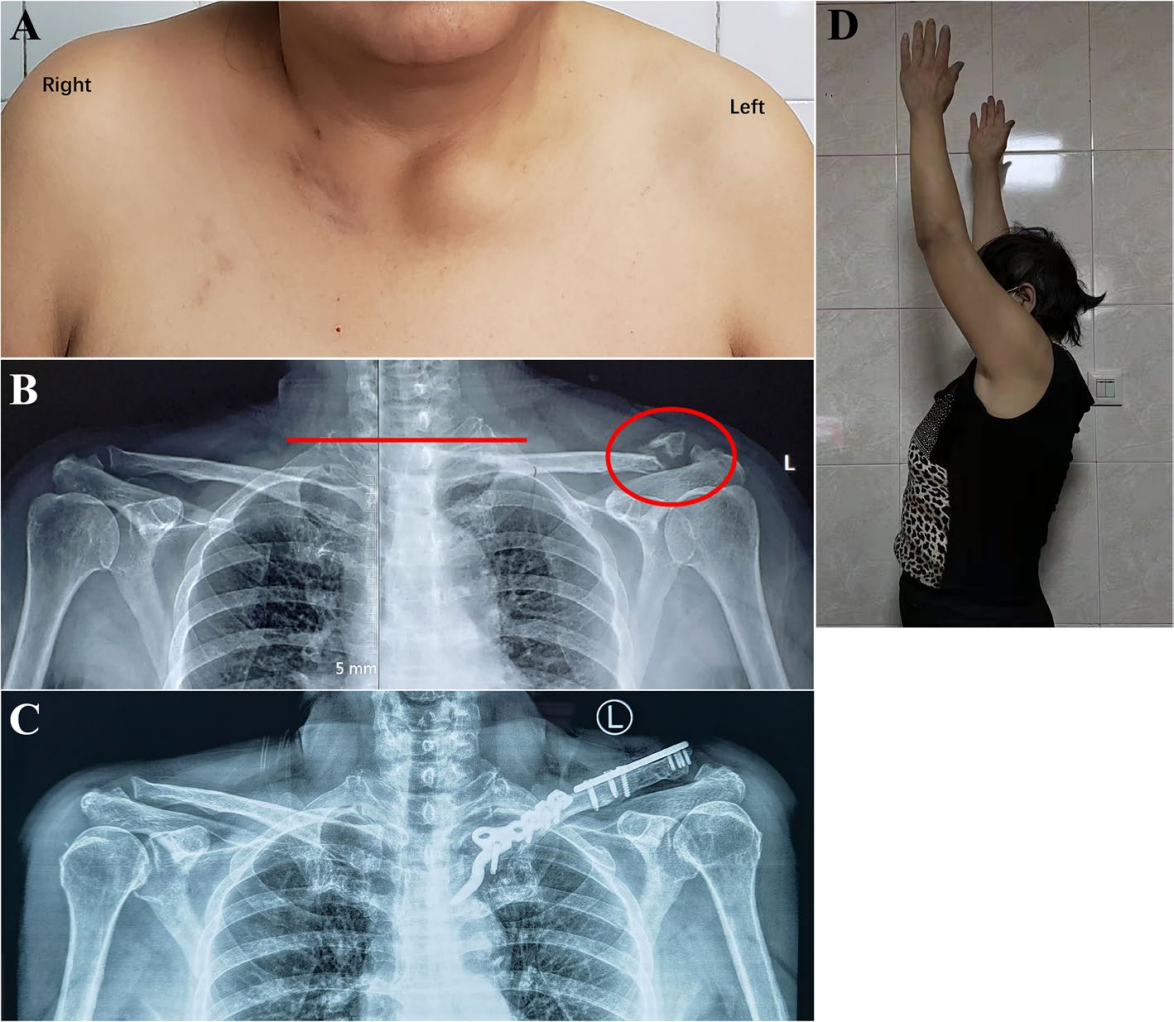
A 58-year-old woman (Patient 3) was injured in a car accident. After repair of the life-threatening injury, she presented to our institute for surgical intervention because of intolerable pain in her shoulder. A An anterior view radiograph showed anterior dislocation of the left sternoclavicular joint. B A preoperative radiograph showed left anterior dislocation of the sternoclavicular joint (SCJ) and an ipsilateral Neer type II distal clavicle fracture. At the 37-month follow-up, C a plain radiograph showed normal alignment of the SCJ, solid union of the distal clavicle, and slight persistent acromioclavicular joint subluxation. D Although she had mild shoulder function limitation, she was able to return to her preinjury job
